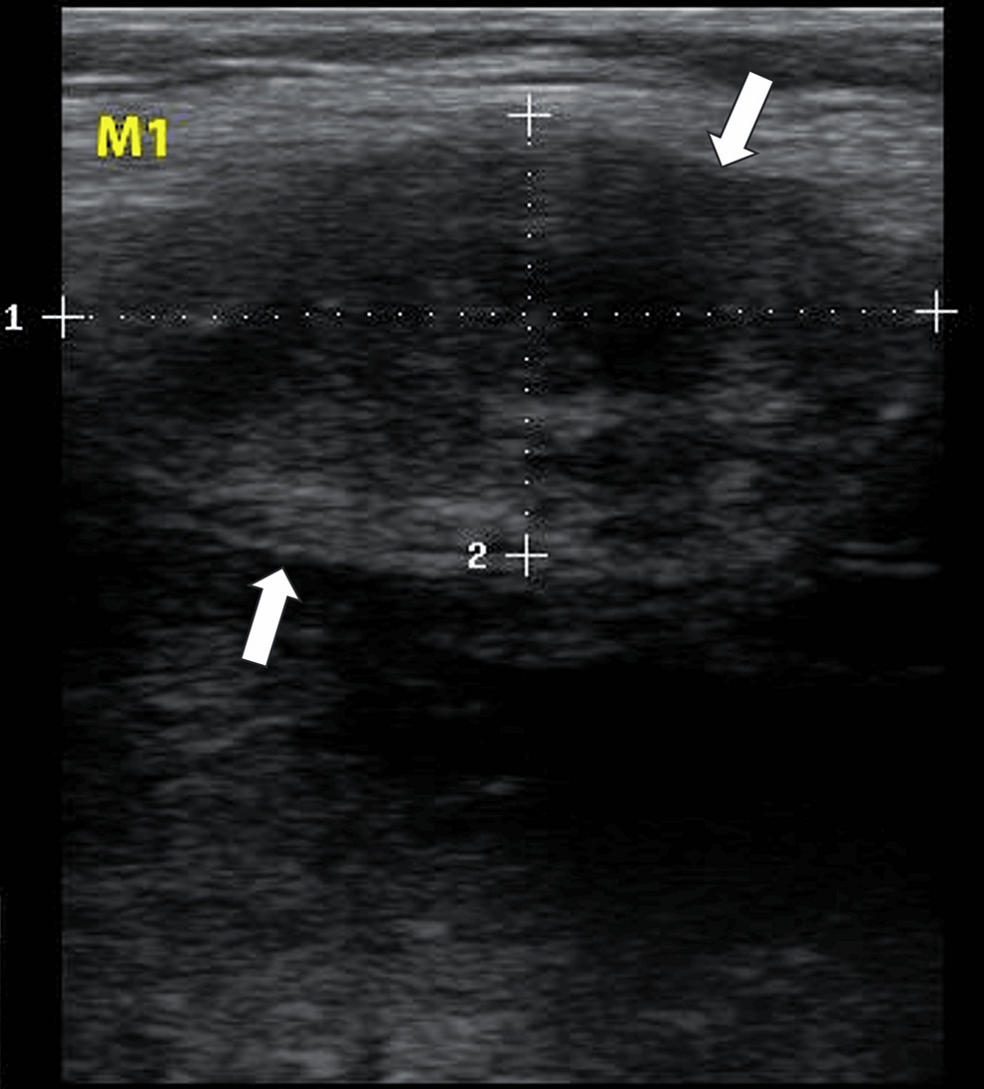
Ultrasound image of M1 The image shows a mass projecting from the tendinous sheath of the vastus lateralis, measuring 3.73 cm (dotted line 1) x 1.89 cm (dotted line 2)
